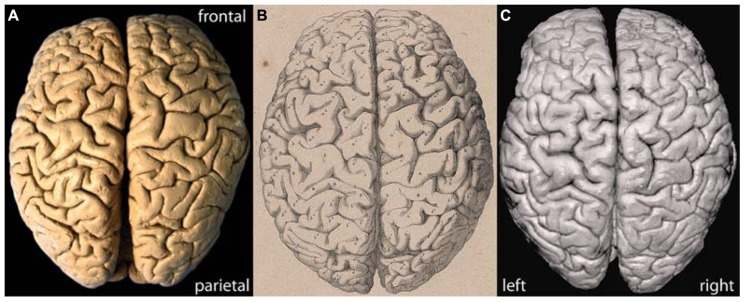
(A) Actual photograph, (B) historic lithograph (Wagner, 1862), and (C) MRI surface reconstruction of the brain of C. H. Fuchs.
